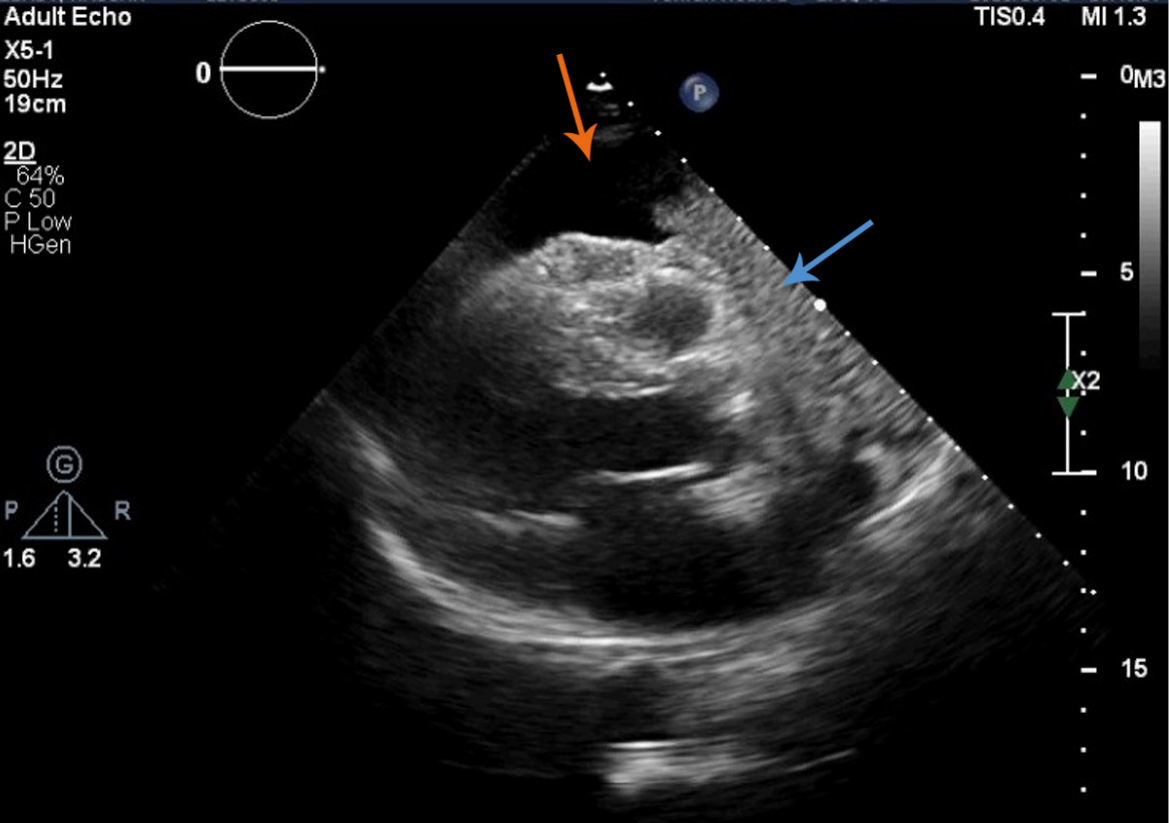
Severe pericardial effusion (orange arrow) and a large mass (blue arrow) anterior to the right ventricular outflow tract in the parasternal long-axis view in 2D transthoracic echocardiography
